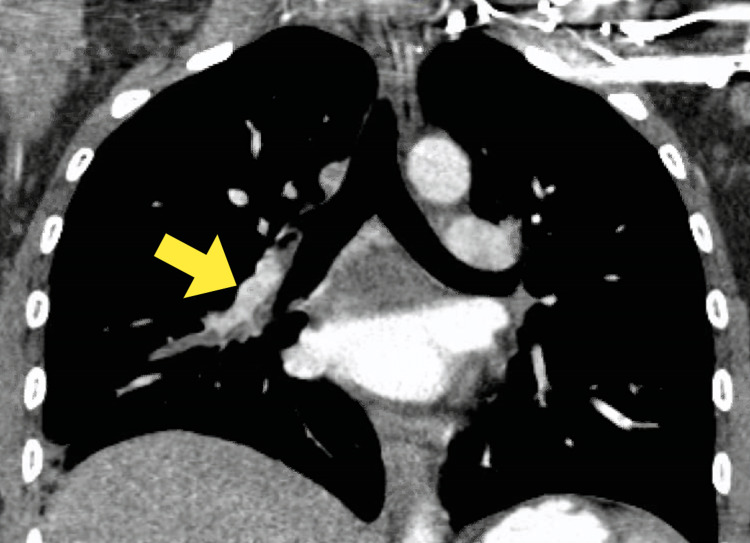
CT scan of the chest showing pulmonary embolism within the segmental and subsegmental branches of the right anterior lung basal arteries
